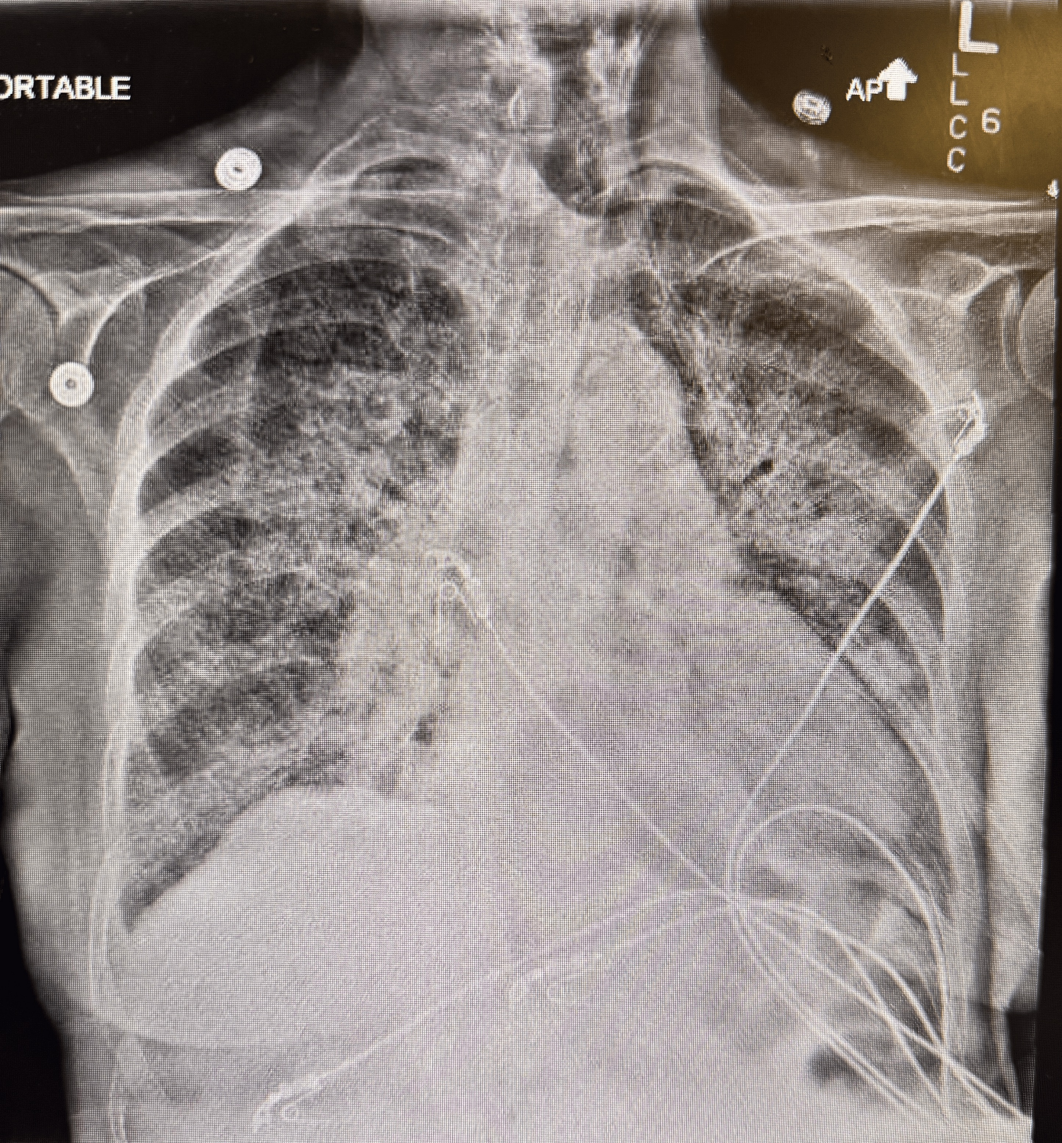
Chest X-ray showing bilateral multiple opacities.
